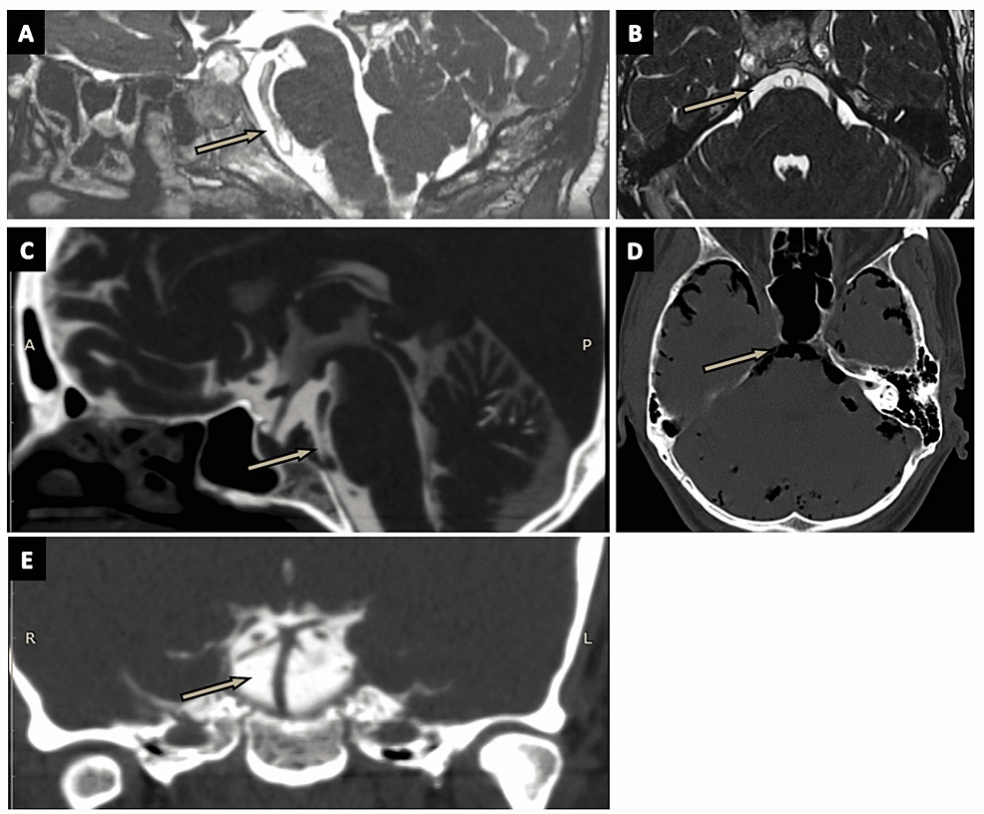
The cisterna pontocerebellaris (prepontine cistern, pontocerebellar cistern). (A) and (B) show the cisterna pontocerebellaris cistern (arrows) in the sagittal and axial sections of the routine three-dimensional (3D) constructive interference in steady state (CISS) MRI sequence, respectively. (C) The cisterna pontocerebellaris (arrow) in the sagittal plane of CT cisternography in a case of suspected CSF rhinorrhea. (D) The cisterna pontocerebellaris (arrow) in the axial section of CT brain (negative contrast) in a case of pneumocephalus. (E) The cisterna pontocerebellaris (arrow) in a coronal section of CT cisternography in a case of suspected CSF rhinorrhea.
